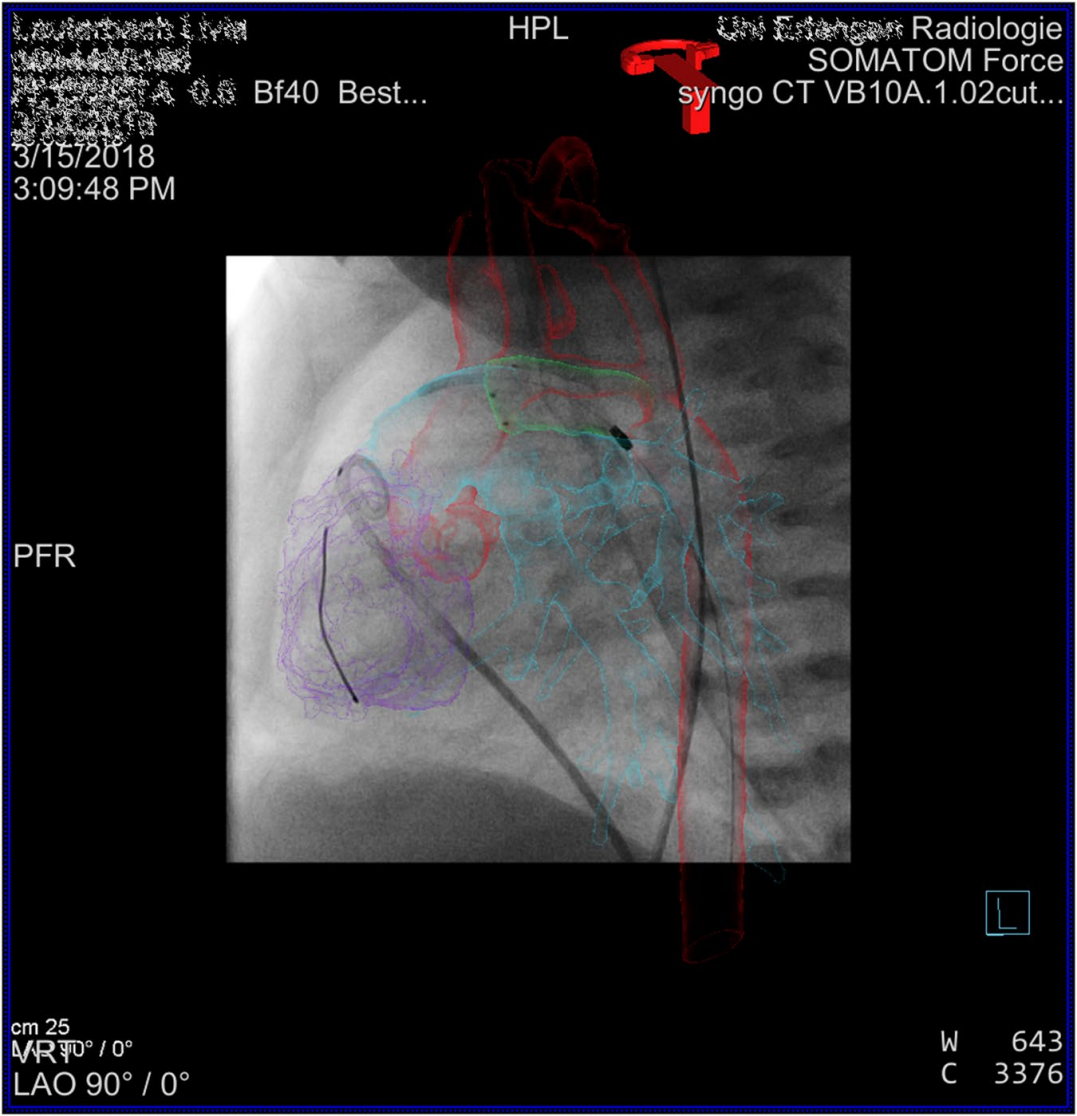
3D-guidance for stenting the arterial duct in a newborn with Hypoplastic left heart syndrome. Basic dataset was a 3^rd^ generation dual source –CT, reconstructed in stereolithographic format. Overlay on both cameras of the biplan angiography machine, shown is the image of the b-camera. The fine delineated model does not obstruct the view on catheters and devices. Color-code light green: ductus arteriosus; red: aorta; violet: right ventricle; light blue: pulmonary artery.
